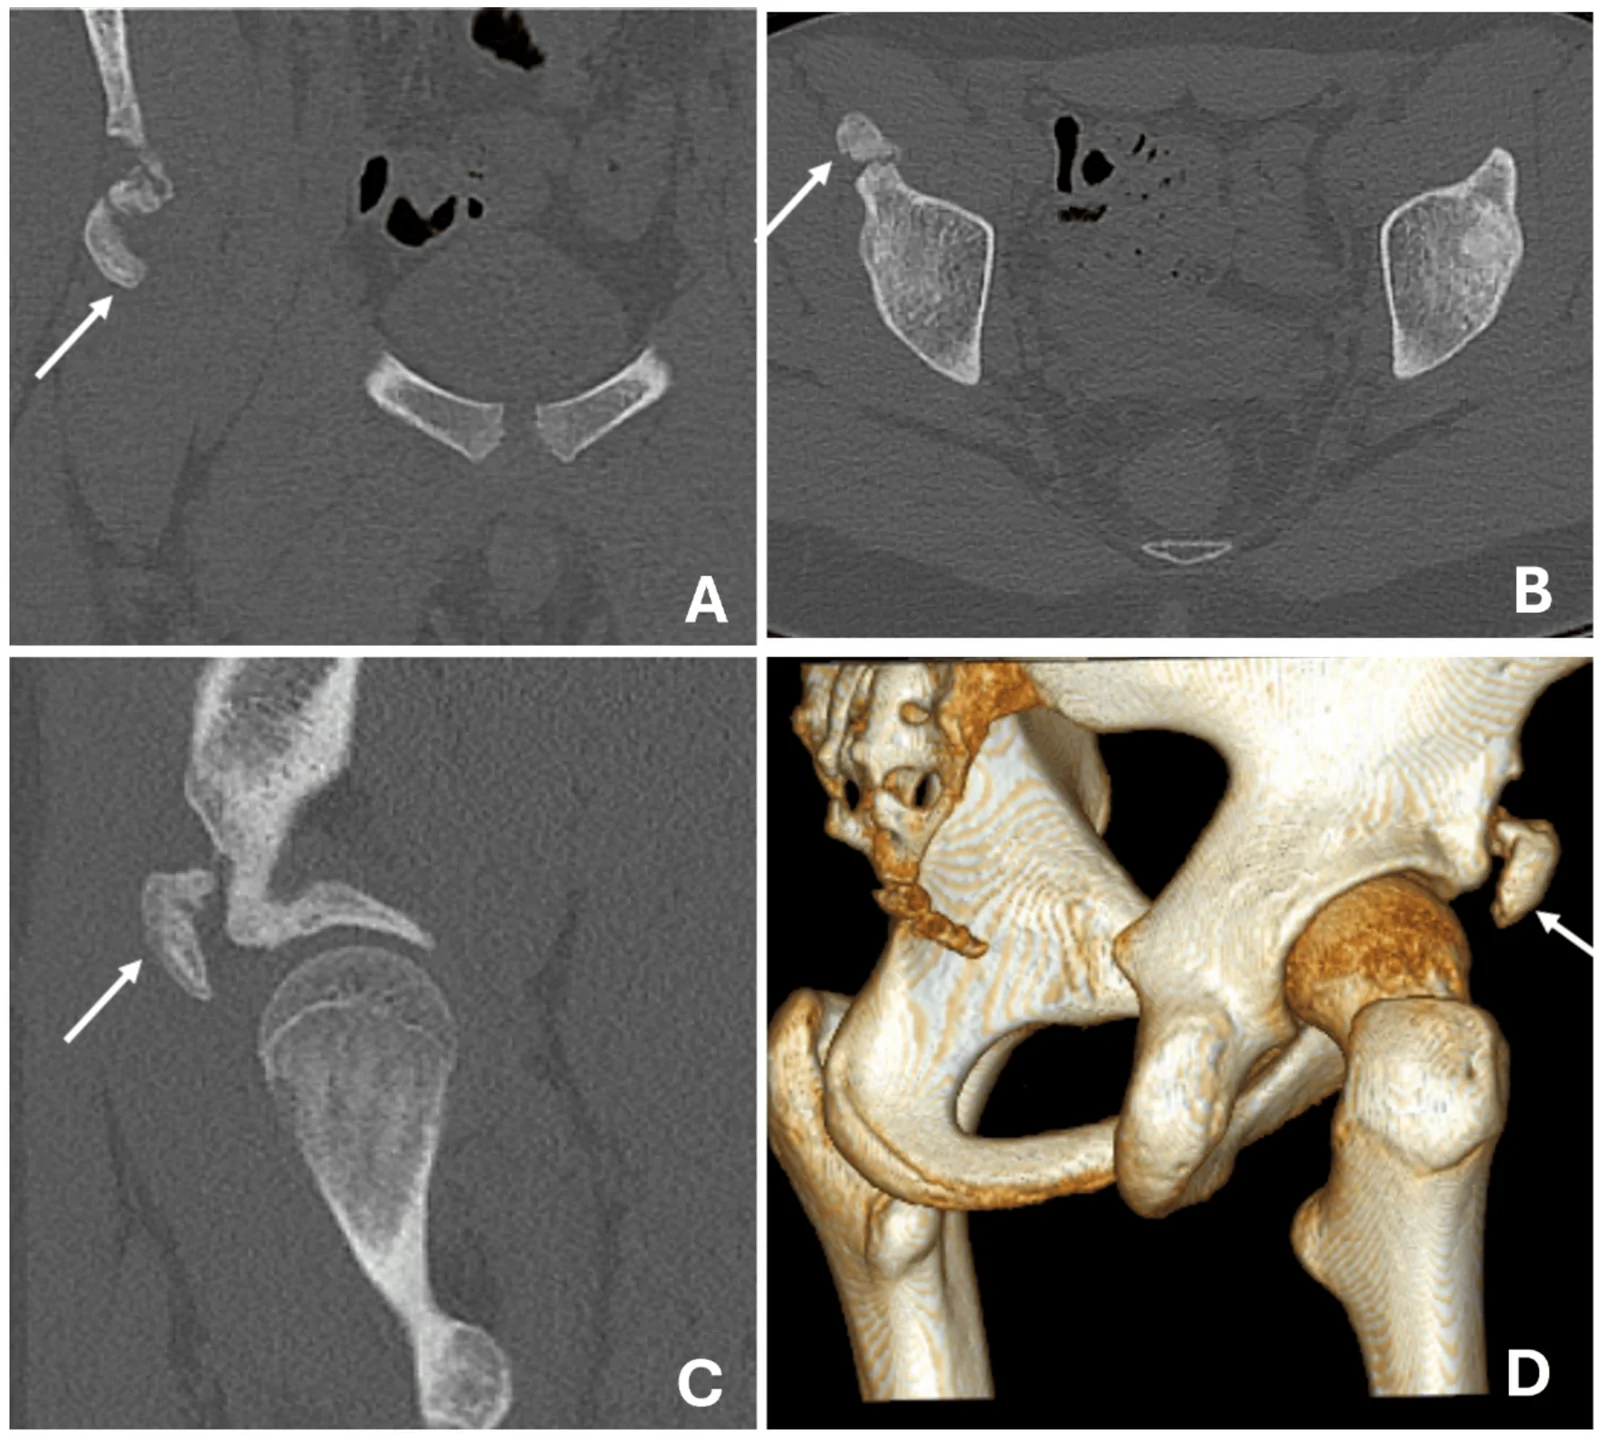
Noncontrast CT of the pelvis. Axial (A), coronal (B), and sagittal (C) planes and 3D VR (D) image confirming the congenital pelvic digit configuration of bony protuberance with changes of pseudoarthrosis with right anterior inferior iliac spine CT: computed tomography; 3D: three dimensional; VR: virtual reality
